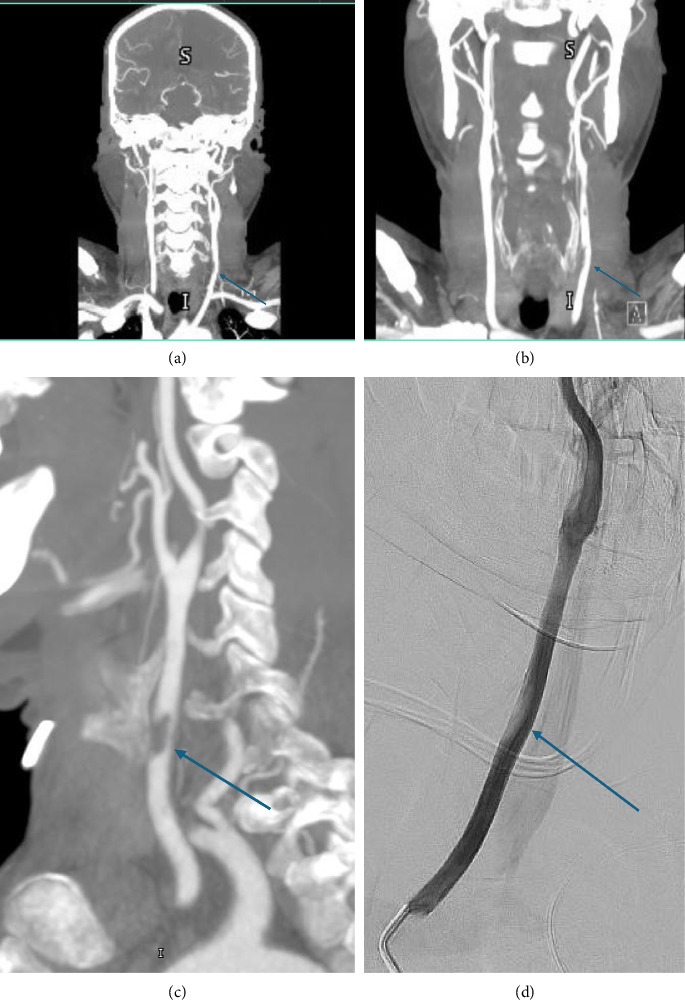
Marked arrows show fresh thrombus at left common carotid artery wall. From left to right (a) and (b) CT angiogram coronal view, (c) CT angiogram sagittal view, and (d) cerebral angiography showing a filling defect indicating a fresh thrombus at left common carotid artery.
